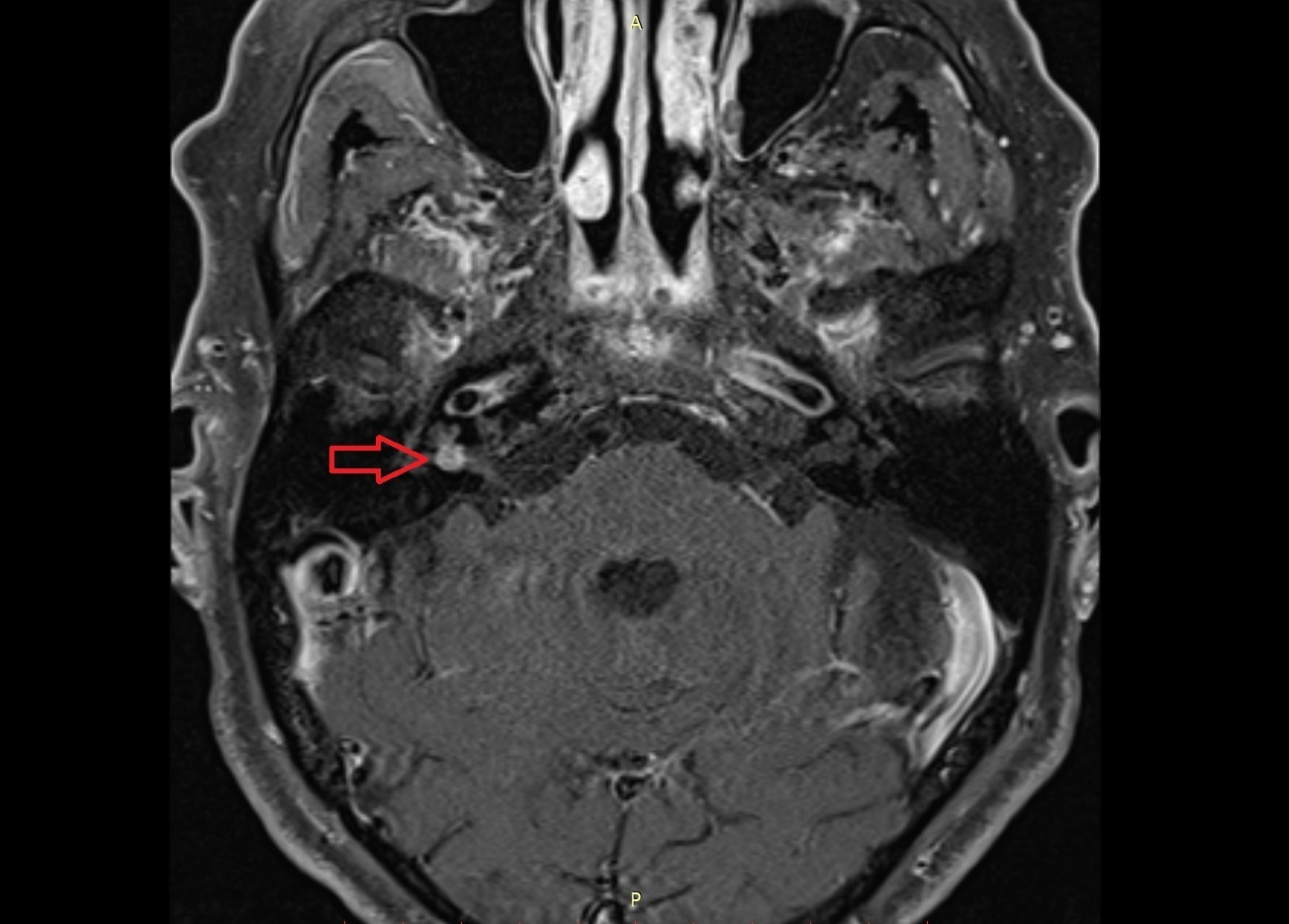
MRI scan. The image shows a round, solitary tumor with a size of 5 x 5 mm inside the right internal acoustic meatus. MRI: magnetic resonance imaging
